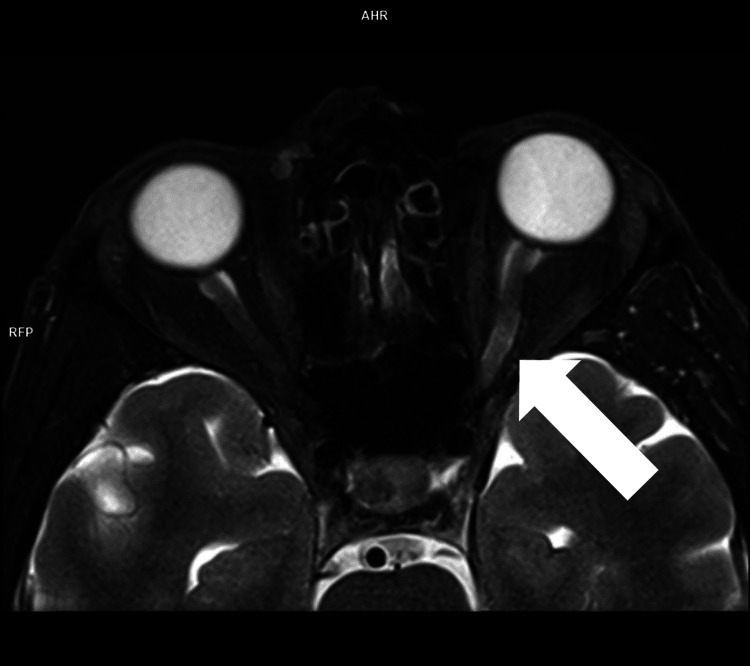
Magnetic resonance imaging (MRI head): transverse T2-weighted sequence White arrow: hyperintensity of the left optic nerve on the transverse T2 Dixon sequence water-only imaging.
